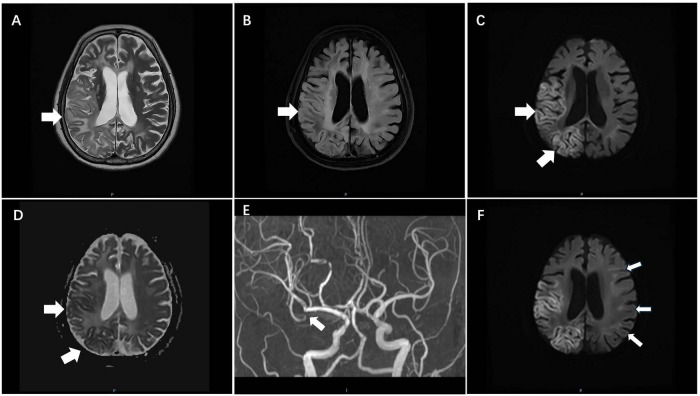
Neuroimaging features of a stroke-like episode in a patient with neuronal intranuclear inclusion disease (NIID). (A) Axial T2-weighted image shows a new, ill-defined area of cortical/subcortical hyperintensity in the right frontal-parietal region (arrow); (B) Fluid-attenuated inversion recovery (FLAIR) sequence confirms the hyperintense lesion in the same region (arrow); (C) Diffusion-weighted imaging (DWI) demonstrates corresponding bright signal (arrow), indicating restricted diffusion; (D) Apparent diffusion coefficient (ADC) map reveals hypointensity (arrow) in the same area; (E) Magnetic resonance angiography (MRA) demonstrates focal stenosis of the right middle cerebral artery M2 segment (arrow); (F) A DWI sequence at a different brain level reveals the pathognomonic sign of NIID: a continuous, linear hyperintensity along the corticomedullary junction (arrow).
